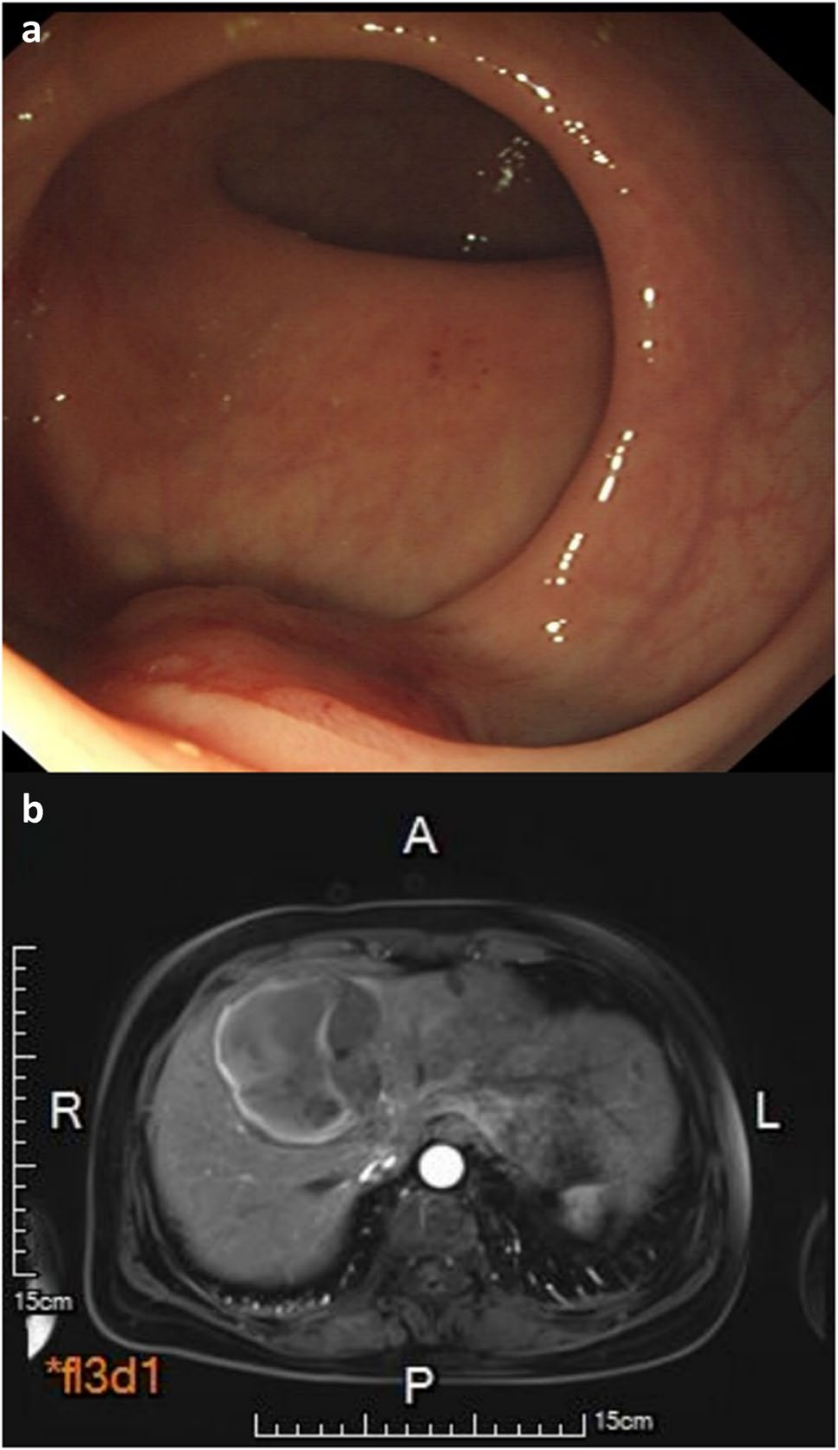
A NET G2, 20 mm in size. a Propelled lesion on the left wall about 3–5 cm from the anus, slightly hyperaemic on the surface mucosa. b MRI indicated multiple liver metastases
